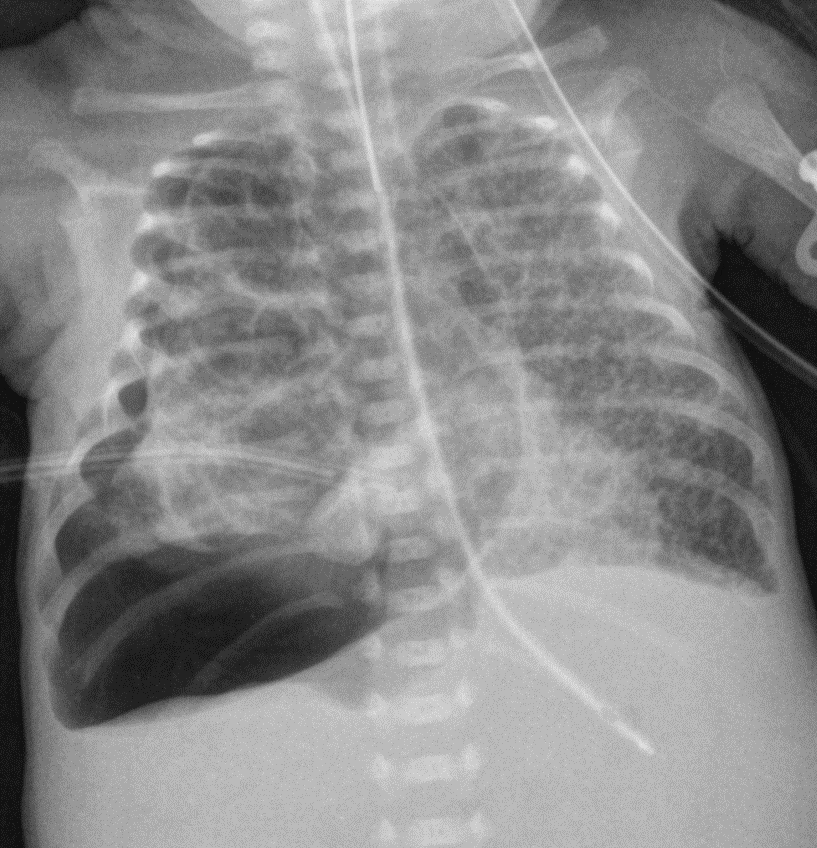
AP chest radiograph 2 days after fibrin glue application shows recurrence of tension right pneumothorax.
